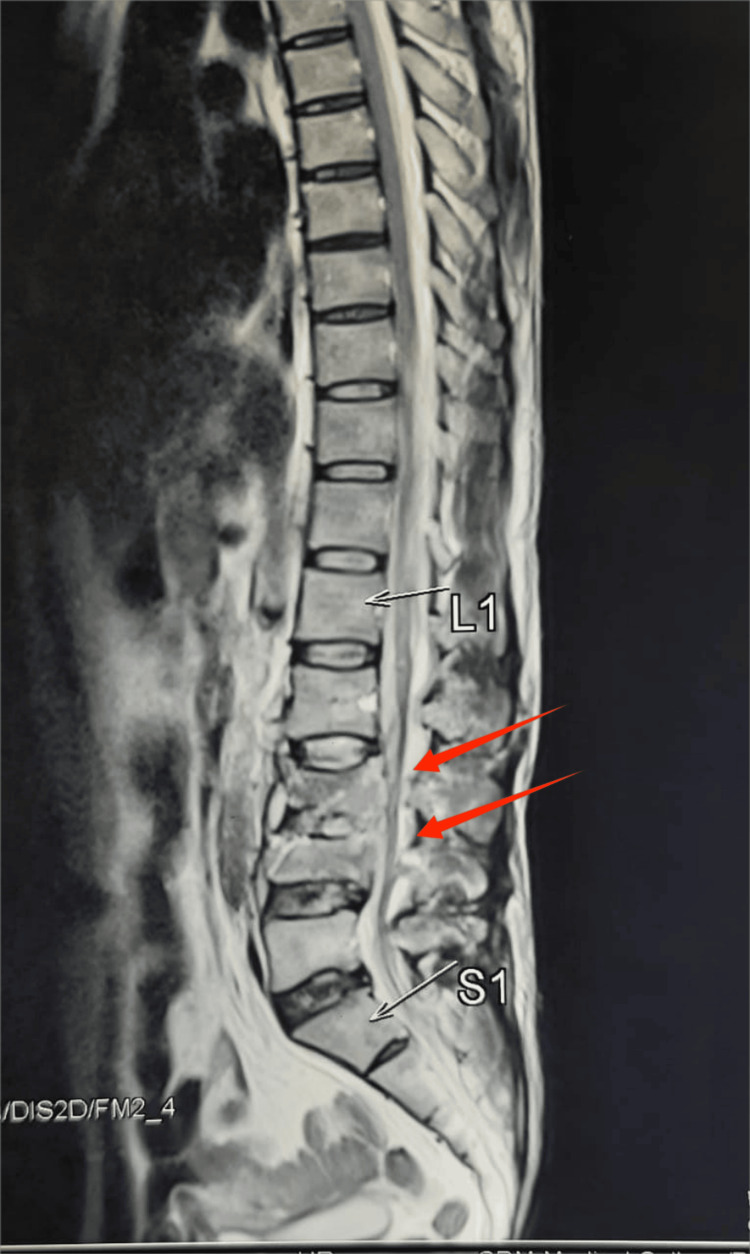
MRI showing L3 and L4 burst fractures with <50% neural canal occlusion MRI, magnetic resonance imaging
